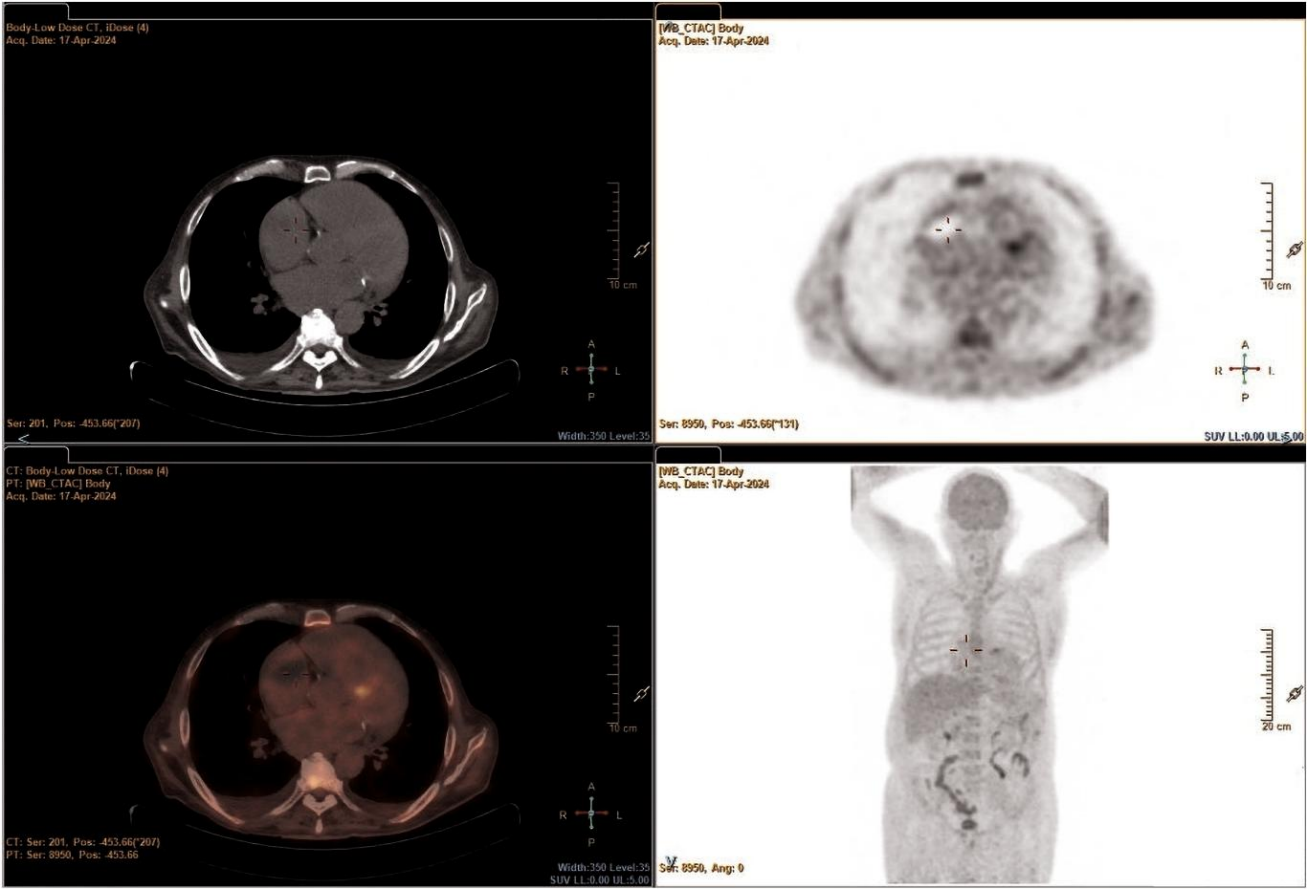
Positron emission tomography/computed tomography scan focused on the heart.
